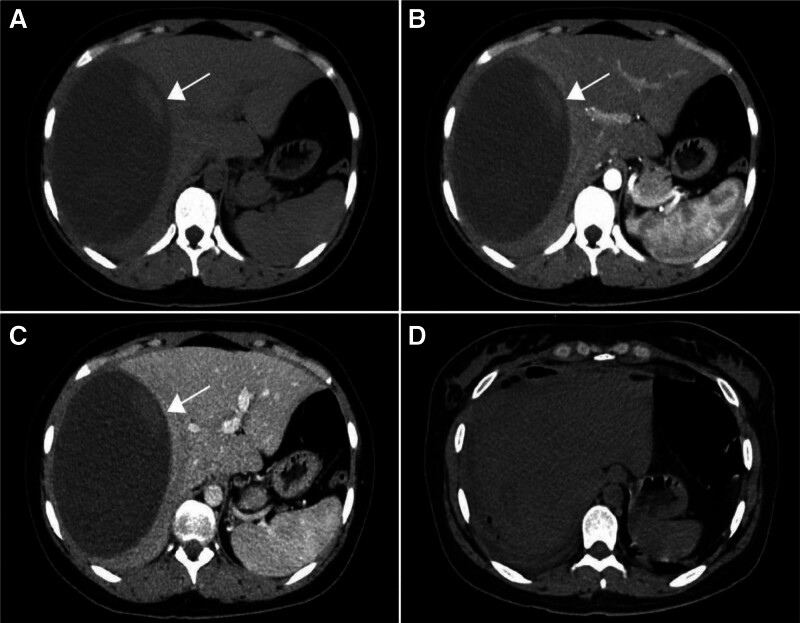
The preoperative plain and enhanced CT images of the patients and the abdominal CT images of the patients were reviewed at 4 months after surgery. (A) CT plain scan showed a 14 × 11.3 cm cystic lesion in the right lobe of the liver, with high-density mass shadow in the lesion. (B) There was no obvious enhancement of the lesion and cyst contents in the arterial phase of CT enhancement. (C) Contrast-enhanced CT showed no significant enhancement of the lesion and cyst contents in the portal vein phase. (D) Four months after the operation, the CT scan showed no obvious abnormality.
